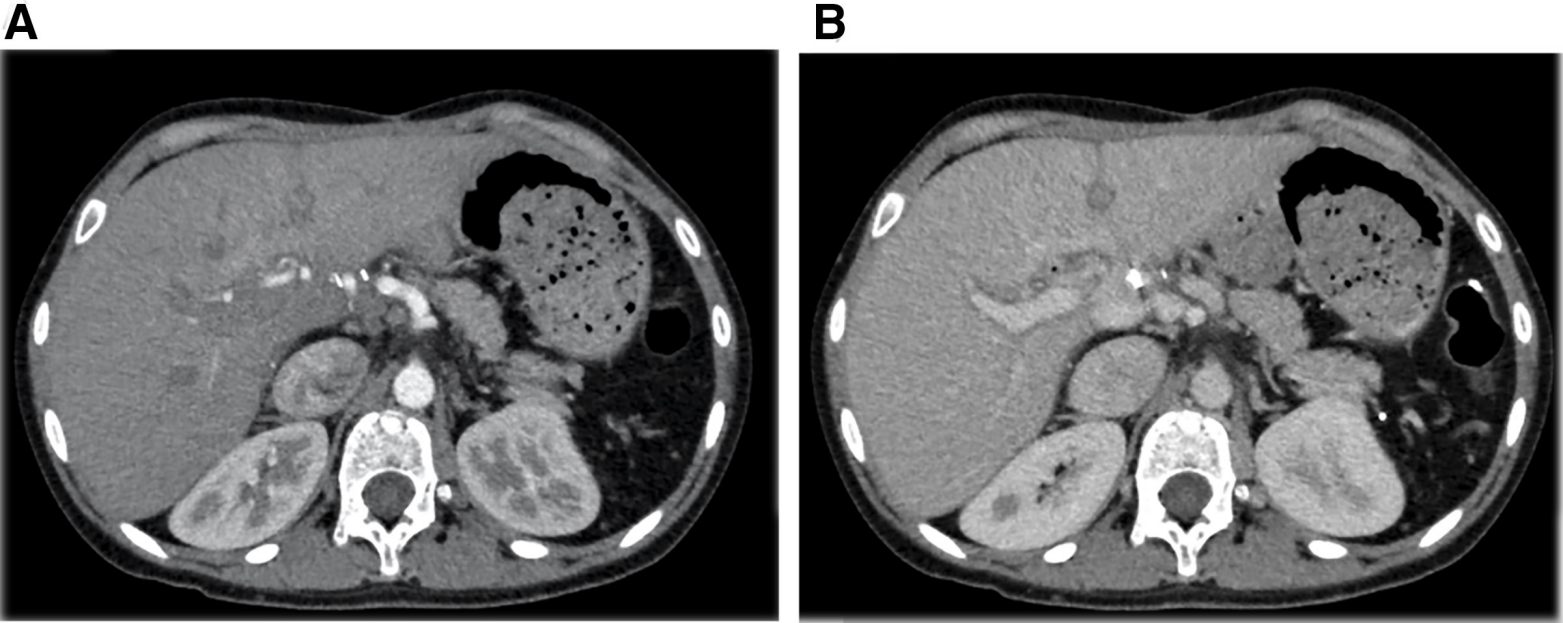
Postoperative CT scan at 3 months after transplantation showing homogeneous enhancement without apparent vascular malformation. (A) Arterial phase and (B) portal phase.
